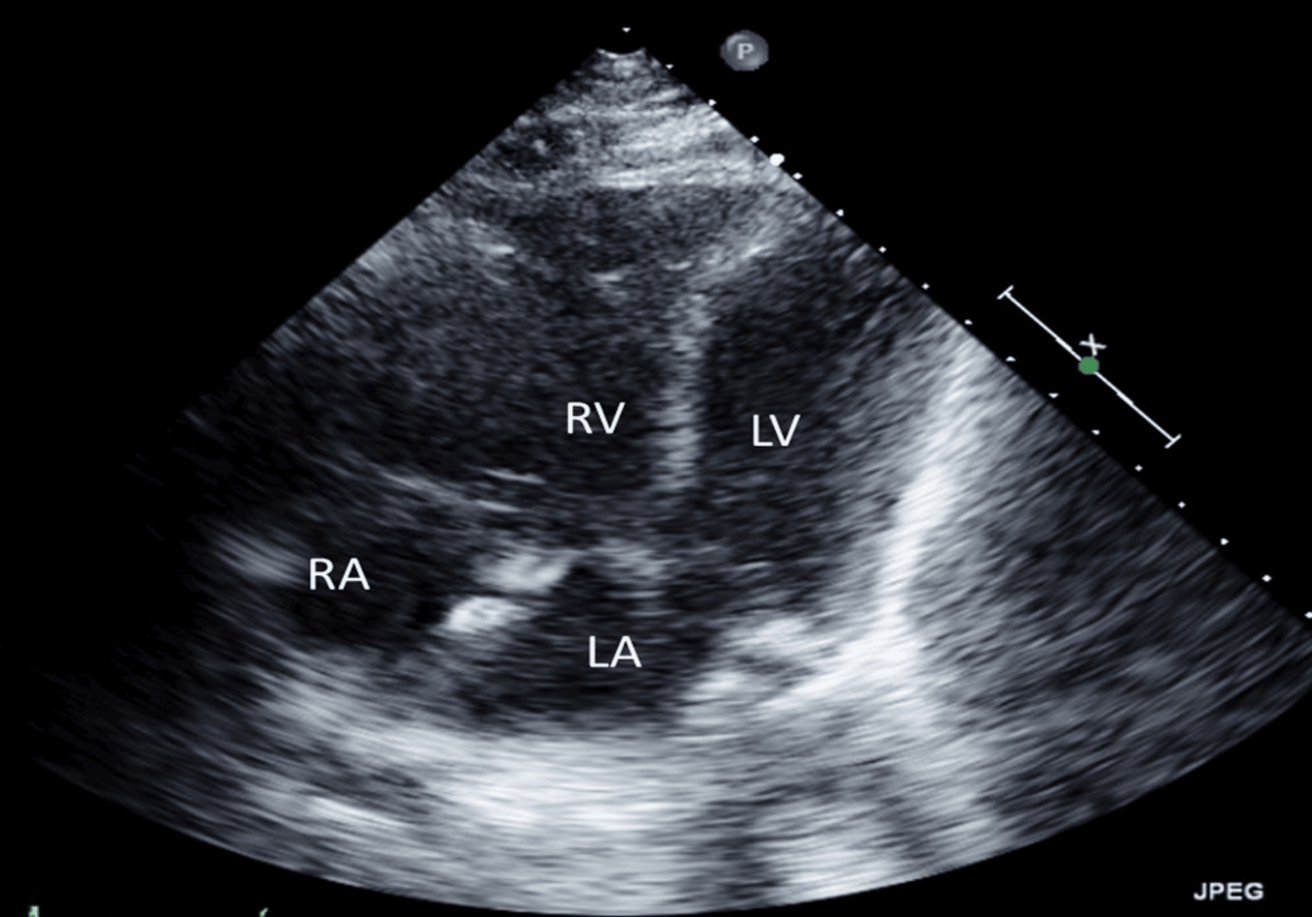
TTE showing chamber view with enlarged RV size and flattening of the septum prior to catheterization TTE: transthoracic echocardiogram, RV: right ventricle, LV: left ventricle, RA: right artery, LA: left artery
